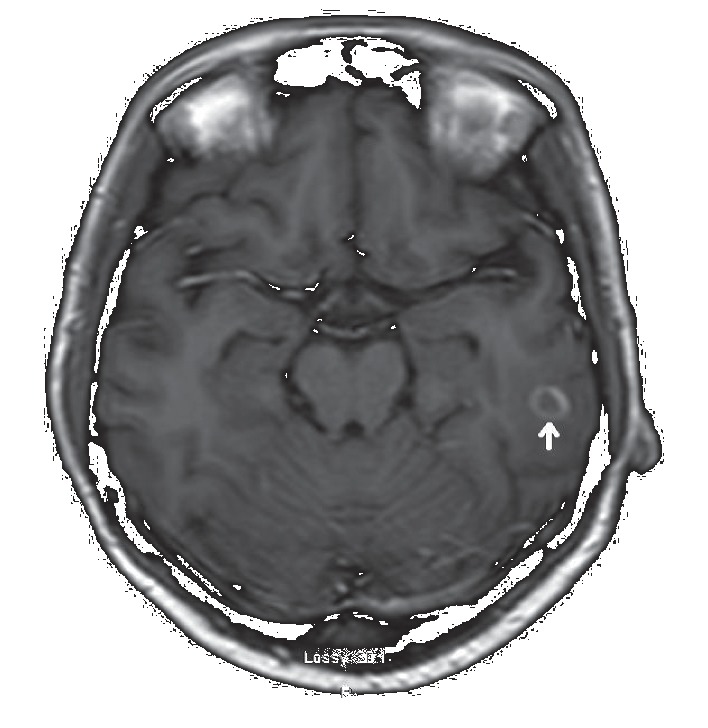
MRI brain showed a peripheral lesion with ring enhancement measuring 1 cm (arrow) in the posterior left temporal lobe with surrounding vasogenic edema without significant mass effect in the ipsilateral basal cistern.
